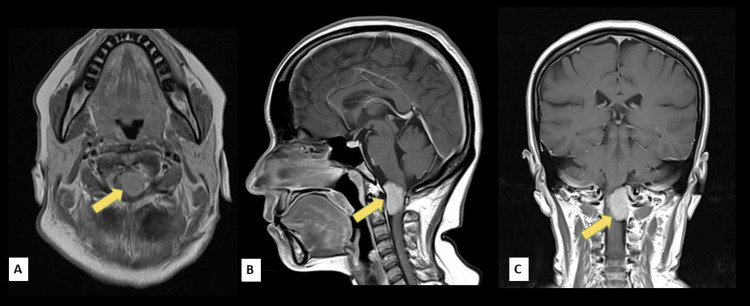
T1-weighted, post-gadolinium cranial magnetic resonance (MR) imaging in (A) axial, (B) sagittal, and (C) coronal views showing a well circumscribed, contrast-enhancing extra-axial mass lesion measuring approximately 2.9x2.1x3.1 cm (CCxWxAP) within the spinal canal at the level of C1-C2, extending from the inferior clivus to the level of the C2 vertebra (arrow) CC: Craniocaudal; W: Width; AP: Anteroposterior
